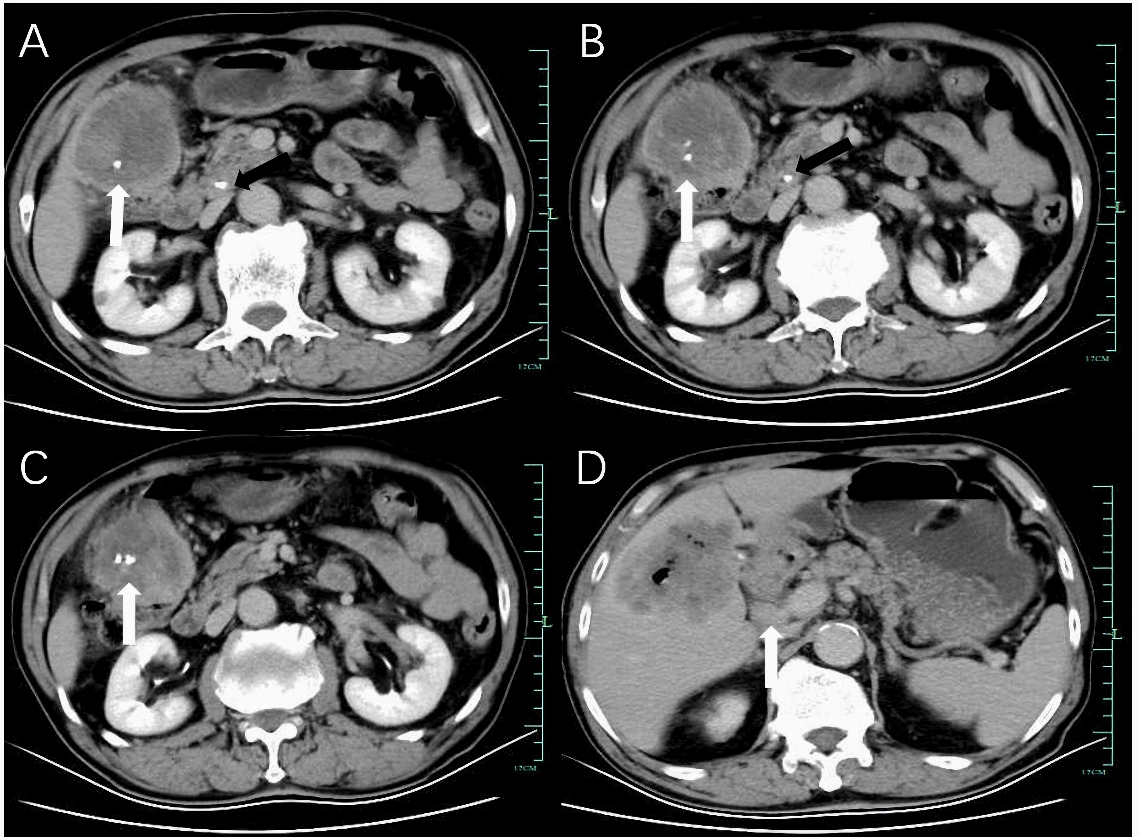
Observations during the equilibrium phase. (A–C) CT images revealed calcifications within the mass (white arrow) and common bile duct stones (black arrow). (D) Enlarged hilar lymph nodes were observed (white arrow).
